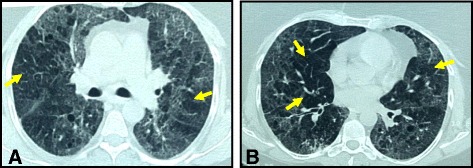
HRCT of HP in a patient: (a) and (b) showing bilateral ground glass opacity with areas of air trapping (mosaic parenchyma) (*arrows*)
